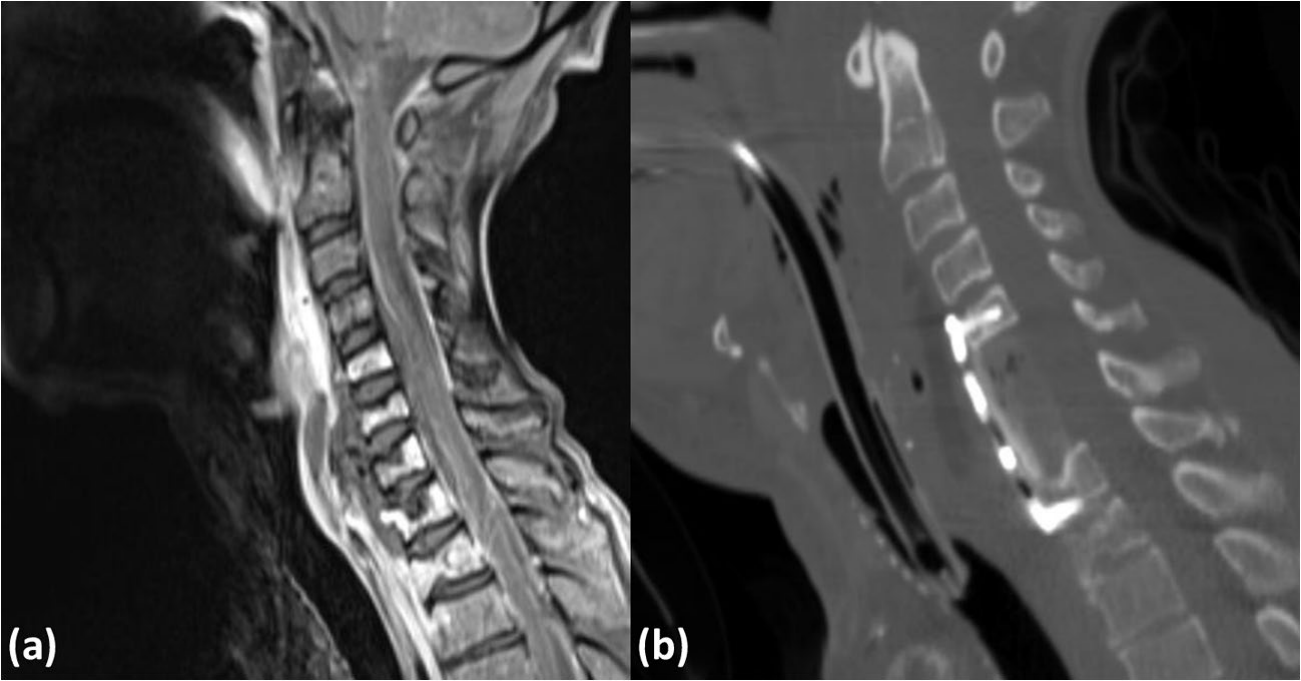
A 54-year-old man with tetraparesis and septic shock. Sagittal MRI (T1-weighted with gadolinium) demonstrates multilevel spondylodiscitis and massive prevertebral and epidural abscess in the lower cervical segments (a). Postoperative CT after emergency surgery including ventral corporectomy of C6 and C7, decompression of the epidural and prevertebral abscess, and fusion with autologous bone graft harvested from the ilium supplemented by plate osteosynthesis (b). The tetraparesis resolved partially prior to discharge for rehabilitation
